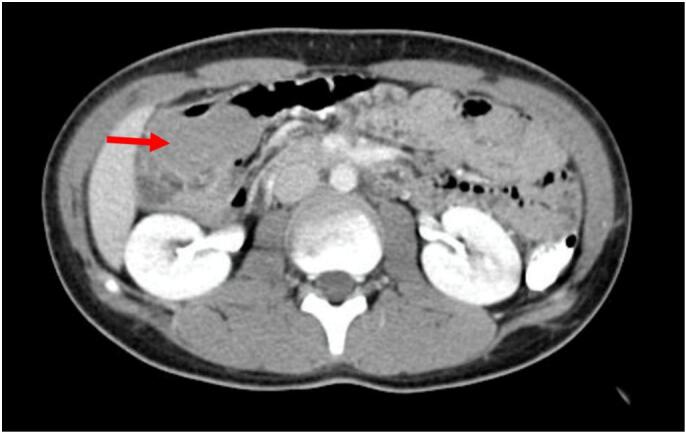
CT scan images showing the intussusception (red arrow).
